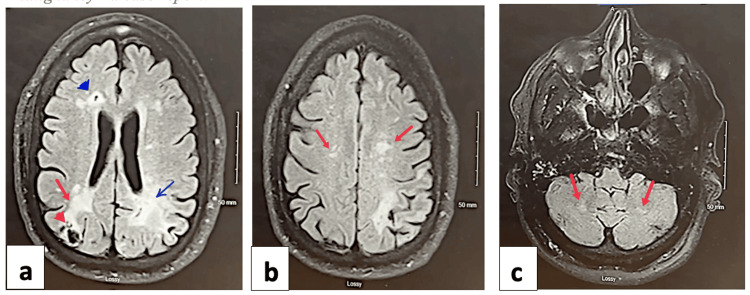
MRI brain – T2 FLAIR axial a) Infarctions in B/L posterior cerebral artery territory R - stable (blue arrow), L - chronic (red arrow) but new from the previous study in a large portion of the left occipital lobe with prior hemorrhagic transformation(red arrowhead); small, chronic, but new inferior frontal gyrus cortical infarction (blue arrowhead); b) B/L chronic unchanged parietal infarctions;(red arrows); c) old lacunar infarctions in cerebellar hemispheres (red arrows)
